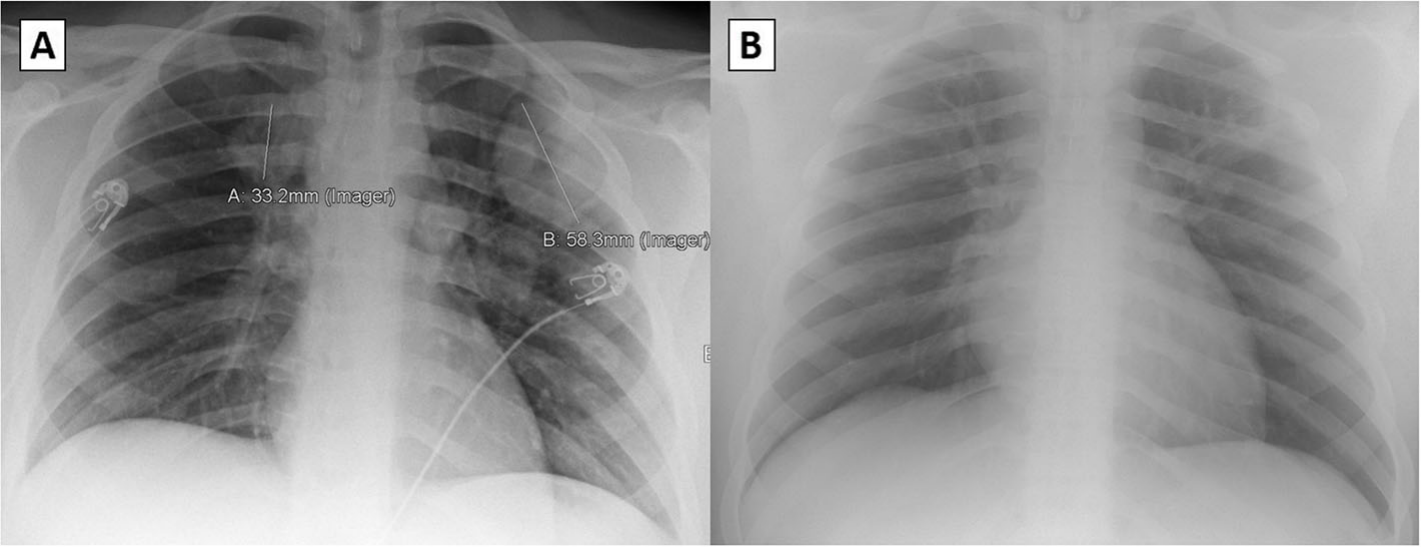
Chest x-ray findings at presentation (A) and 3-month follow-up (B)
